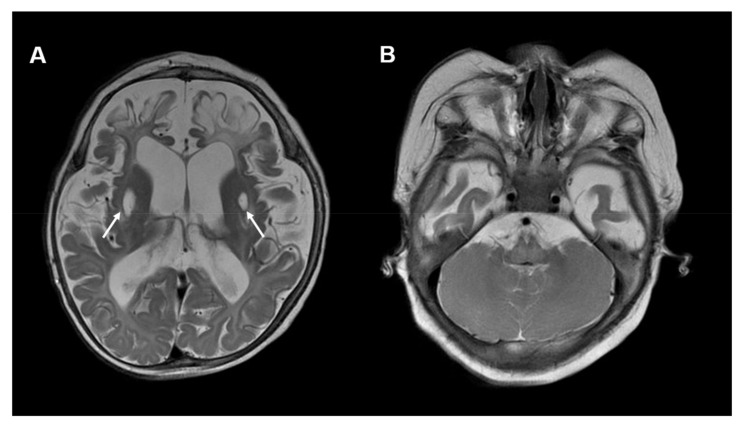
Neuroimaging in COQ7 deficiency: (A) Brain MRI, T2-weighted, axial images of a 10-month-old boy with COQ7 deficiency. The MRI shows global brain atrophy and areas of encephalomalacia in bilateral frontal lobes. In addition, symmetric cystic changes within the putamen are visible (white arrows). (B) No cerebellar abnormalities are visible. Other MRI images of this individual were published previously [41].
